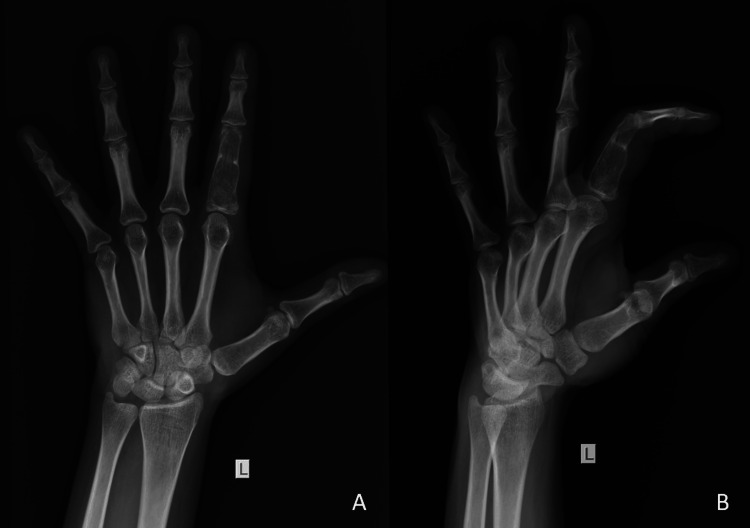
Face (A) and profile (B) x-rays of the hand, five weeks postoperatively
